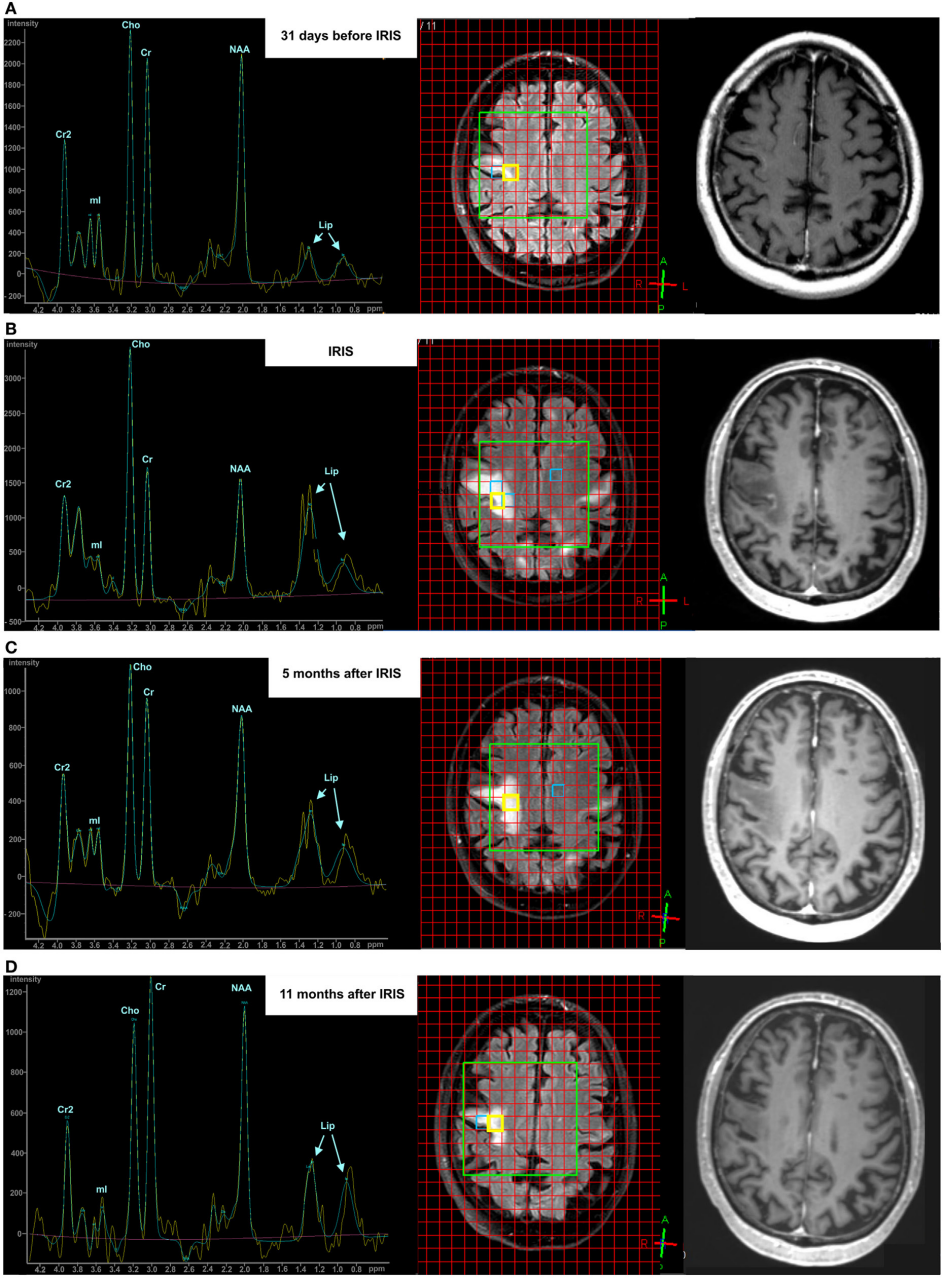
Exemplary course of progressive multifocal leukoencephalopathy (PML) in a single patient visualized by conventional magnetic resonance imaging (fluid-attenuated inversion recovery-sequence and Gadolinium-enhanced T1 sequence) and ^1^H-MRS during the pre-immune reconstitution inflammatory syndrome (IRIS) phase [(A) upper row], IRIS phase [(B) middle row], 5 months post-PML phase [(C) third row], and 11 months post-PML phase [(D) lower row].
